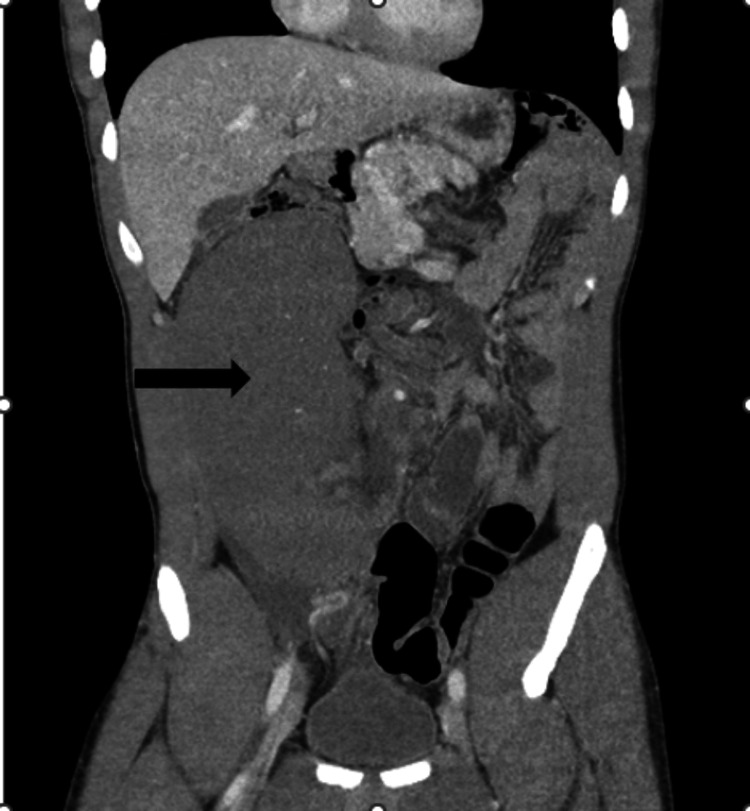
CT abdomen and pelvis, coronal view, slice 27. Black arrow representing wandering spleen.
